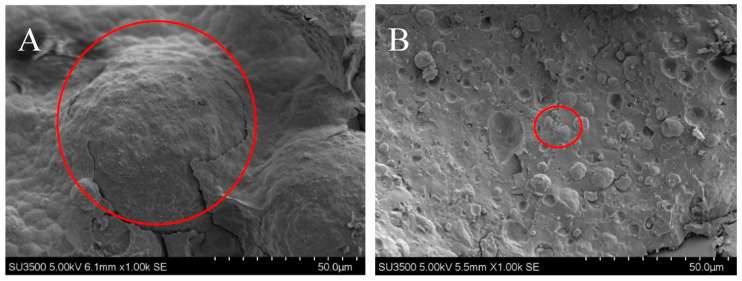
The Cryo-SEM images of the 1:10 emulsions before (A) and after (B) high-intensity ultrasound emulsification. The spherical objects in the red circle represent emulsion droplets.
